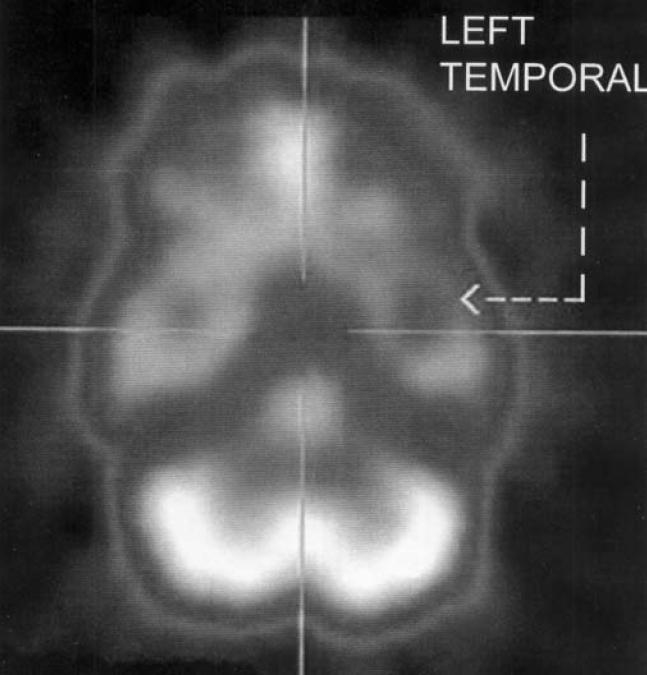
SPECT scan showing reduced perfusion in the left temporal lobe in subject no. 3
